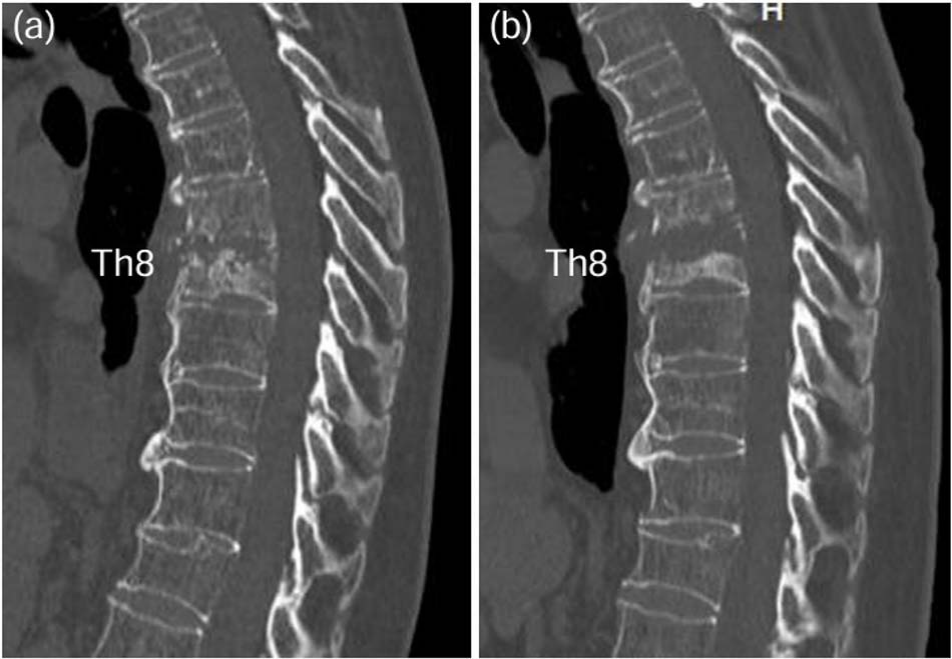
CT scan revealed osteolysis of the Th7-Th8 vertebral endplates and DISH upon admission (a). Four weeks later, progression of destructive changes at the Th7-Th8 endplates was observed (b).
